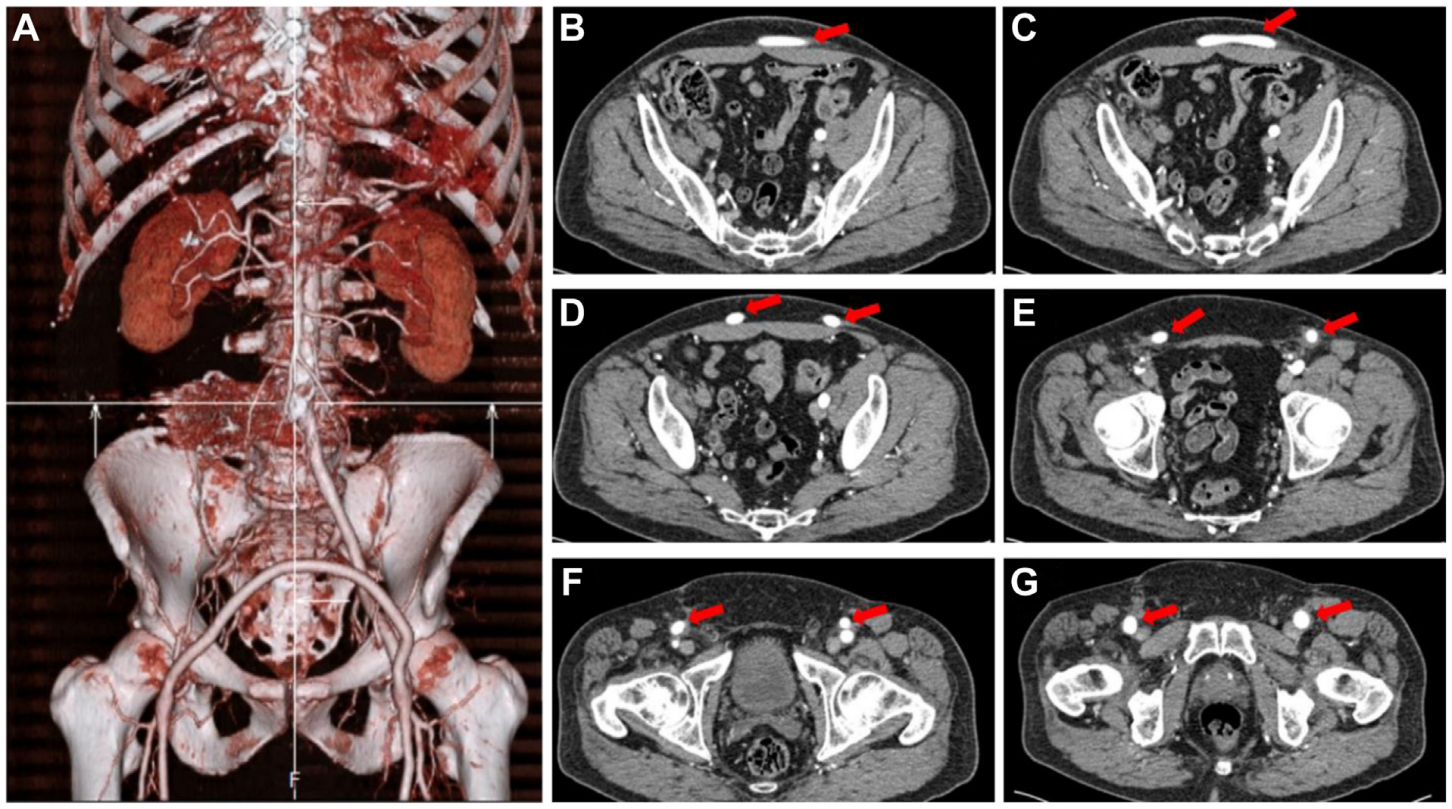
Bypass reconstruction and flow. (A) A three-dimensional computed tomography reconstruction of the patient’s bypass graft 6 months post operation. (B-G) Computed tomgraphy angiogram (CTA) of blood flow through the bypass graft. *Red arrows* correspond with the bypass graft until it merges into the femoral arteries bilaterally.
